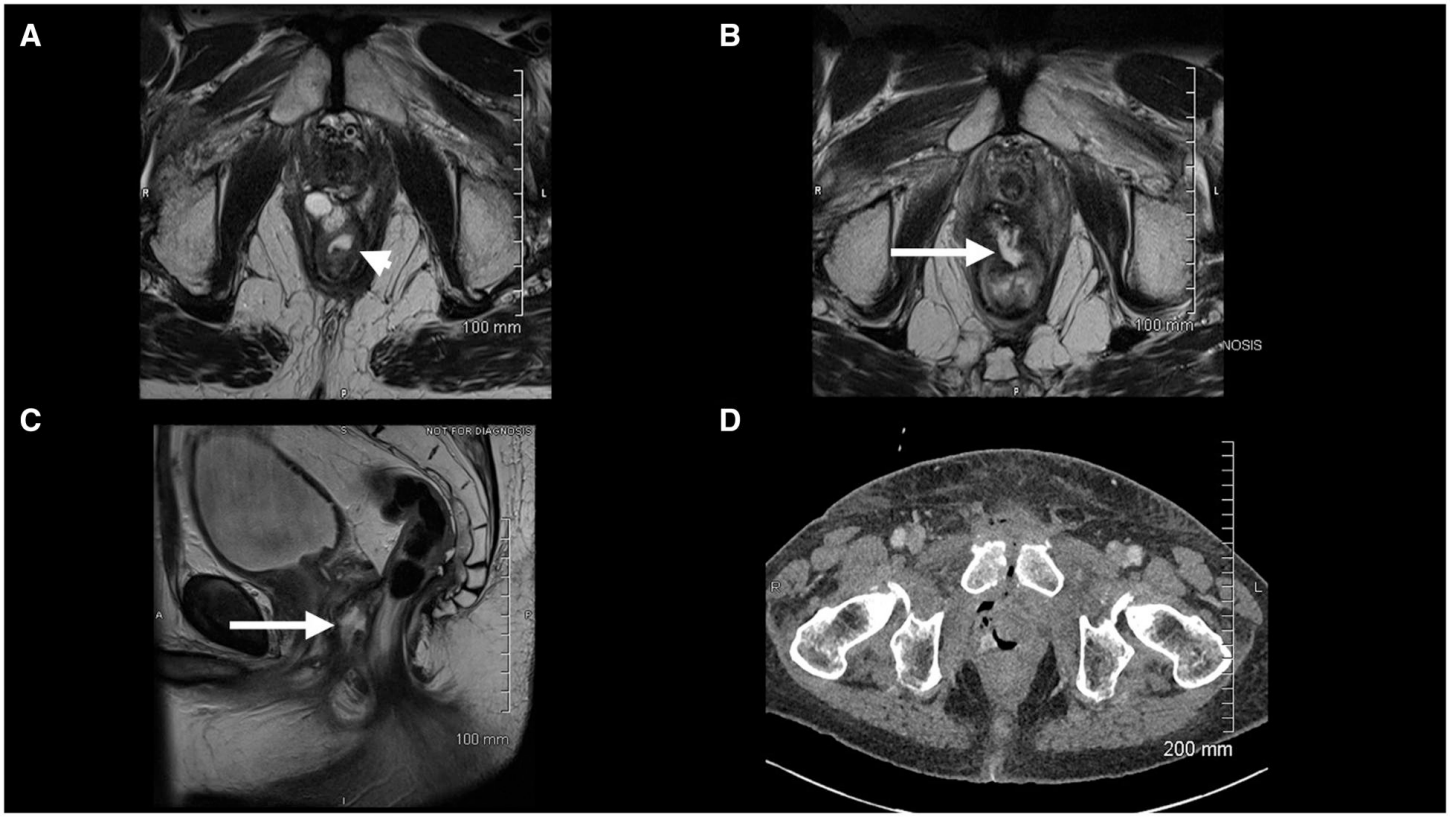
(A) Selected axial T2 weighted images from an MRI pelvis after hydrogel injection and before radiation therapy showing a collection within the anterior rectal wall (arrow head). (B and C) Selected axial and sagittal images from an MRI pelvis after radiotherapy treatment showing an enlarging defect in the anterior rectal wall communicating with a collection between the rectum and the prostate in keeping with an abscess (arrow). (D) Selected axial image from a CT abdomen and pelvis with intravenous contrast showing the collection containing fluid and air to track anteriorly in the pelvis and involve the pubic symphysis with cortical erosion consistent with osteomyelitis.
